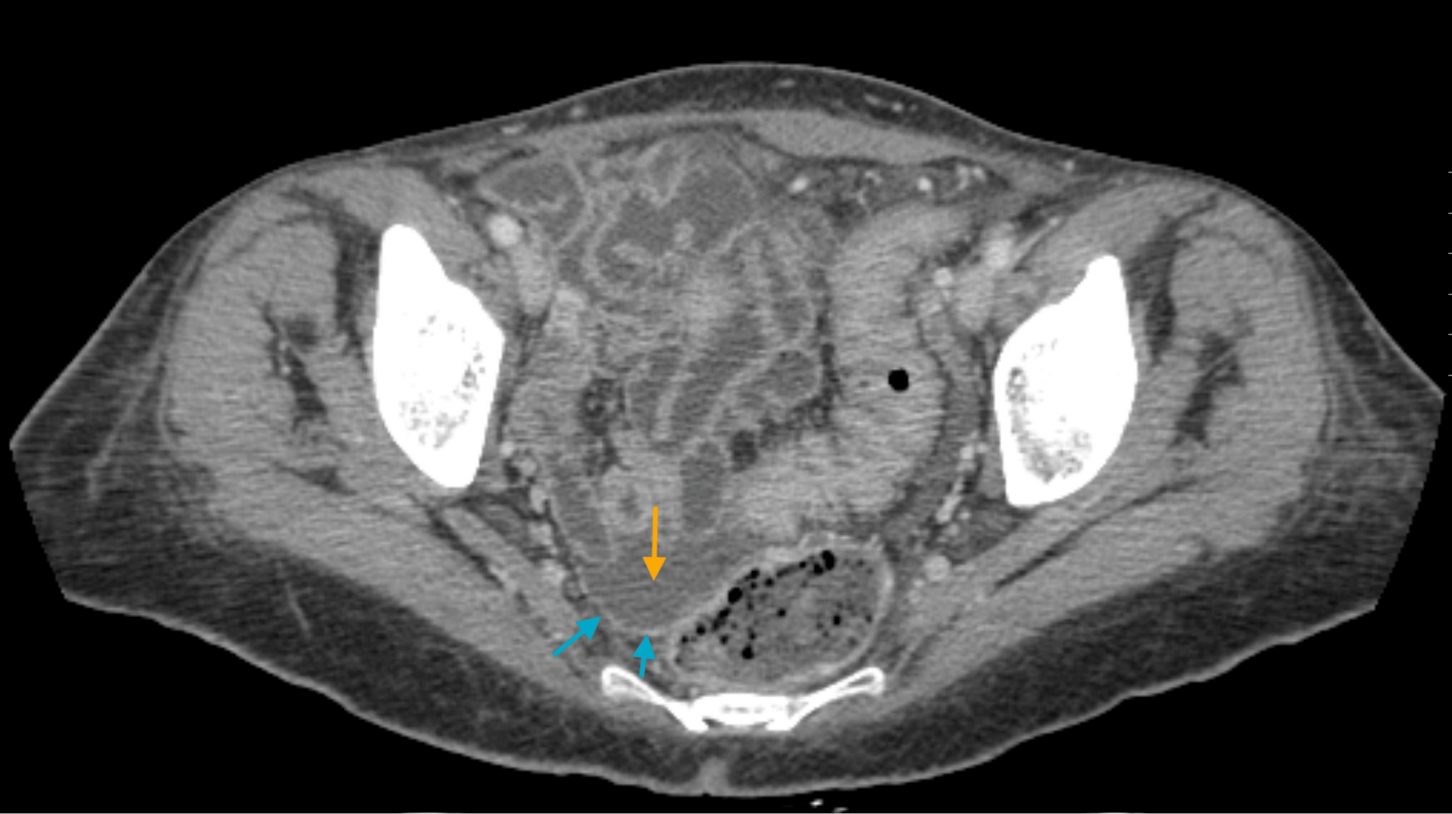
Axial CT scan of the pelvis showing irregular peritoneal thickening (blue arrows) and a small amount of peritoneal fluid (yellow arrow), findings suggestive of peritoneal carcinomatosis
